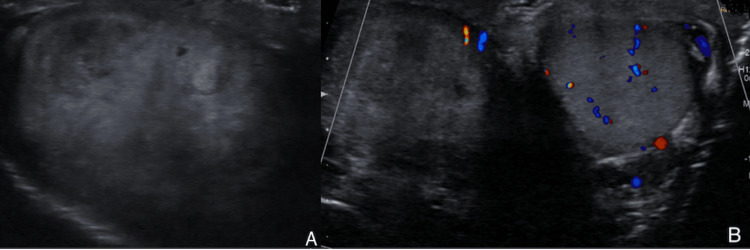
Scrotal ultrasound of an enlarged 5.4 cm diffusely heterogenous right testicle (A) and Doppler ultrasonography with minimal spectral Doppler flow indicating right testicular torsion (B)
